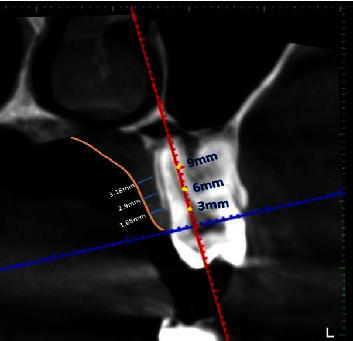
CBCT-coronal view shows each point that was marked from the cementoenamel junction with a 3 mm interval (yellow dots) along a line bisecting the palatal root as a reference. A perpendicular line (blue line) joins these points to the tangential line drown (pink line).
